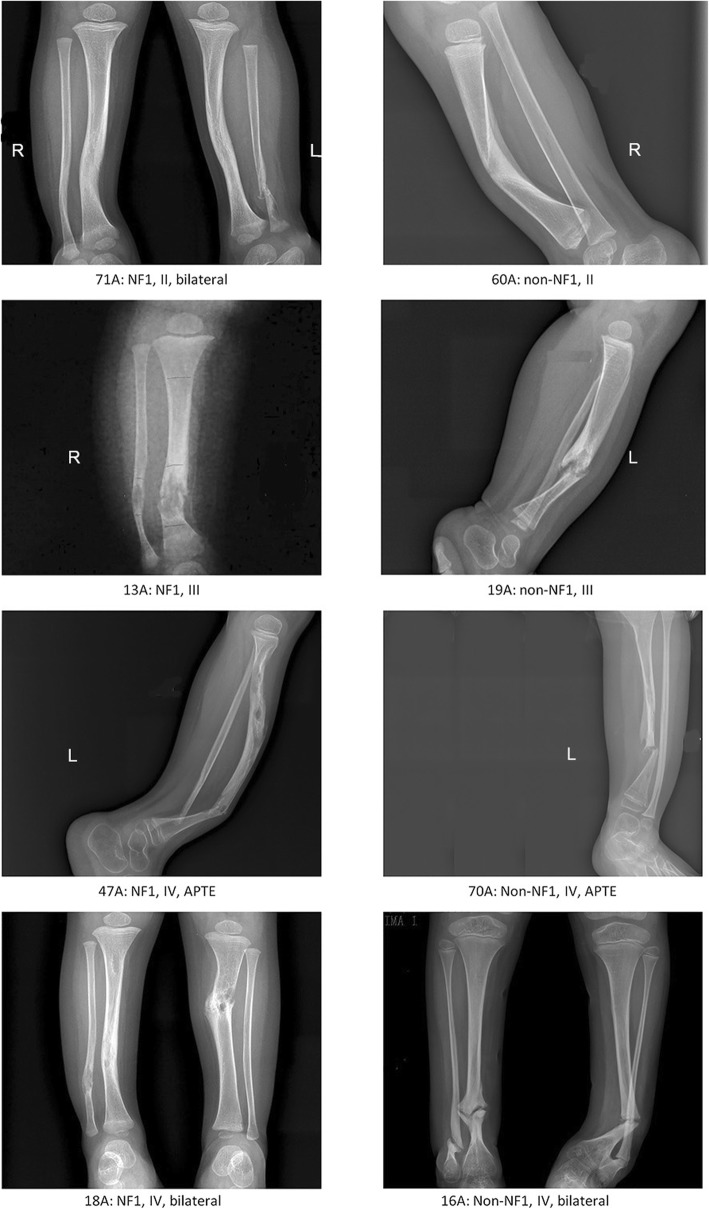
X-ray images of four NF1 CPT vs. four non-NF1 CPT patients. Four NF1 CPT patients show at the left column, and four non-NF1 CPT patients show at the right column. Case 71A (NF1) and 60A (non-NF1) are Crawford II type showing cortical thickening and narrowed medullary canal; case 13A (NF1) and 19A (non-NF1) are Crawford III type with cystic lesion; case 47A (NF1) and 70A (non-NF1) were Crawford IV type presenting pseudarthrosis and an abnormality of the proximal tibial epiphysis (APTE); case 18A (NF1) and 16A (non-NF1) are bilateral and are classified as Crawford IV type
